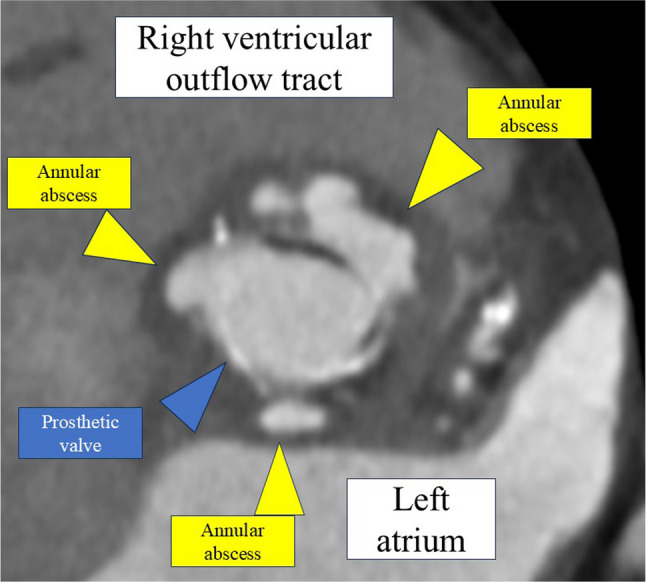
Contrast-enhanced computed tomography showing annular abscess formation. Contrast-enhanced computed tomography at the level of the aortic root reveals multiple low-attenuation lesions around the prosthetic valve consistent with annular abscess formation (yellow arrowheads). The prosthetic valve is indicated (blue arrowhead), and surrounding anatomical structures, including the right ventricular outflow tract and left atrium, are labeled
